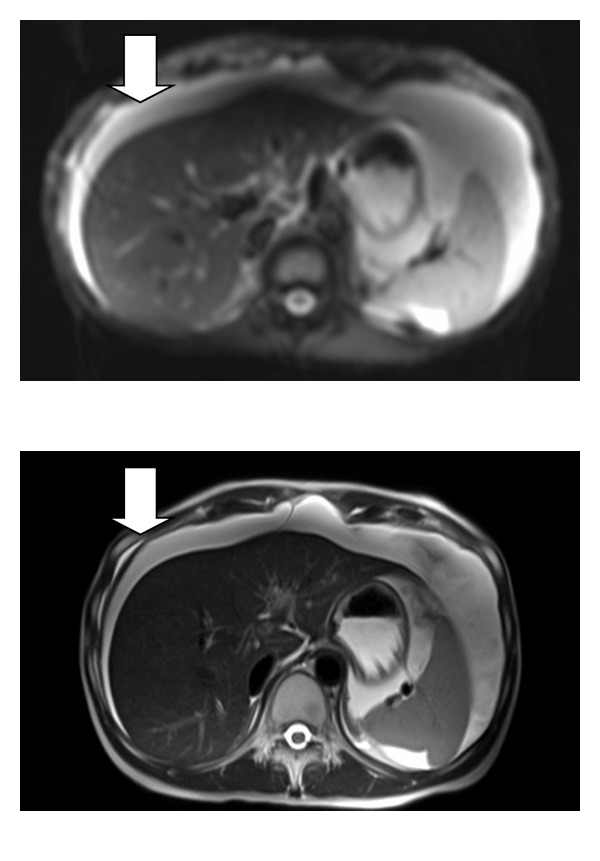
Diffusion-weighted MRI demonstrating ascites (arrow) in patients with gastric cancer following partial gastrectomy with chemotherapy-associated steatohepatitis.
